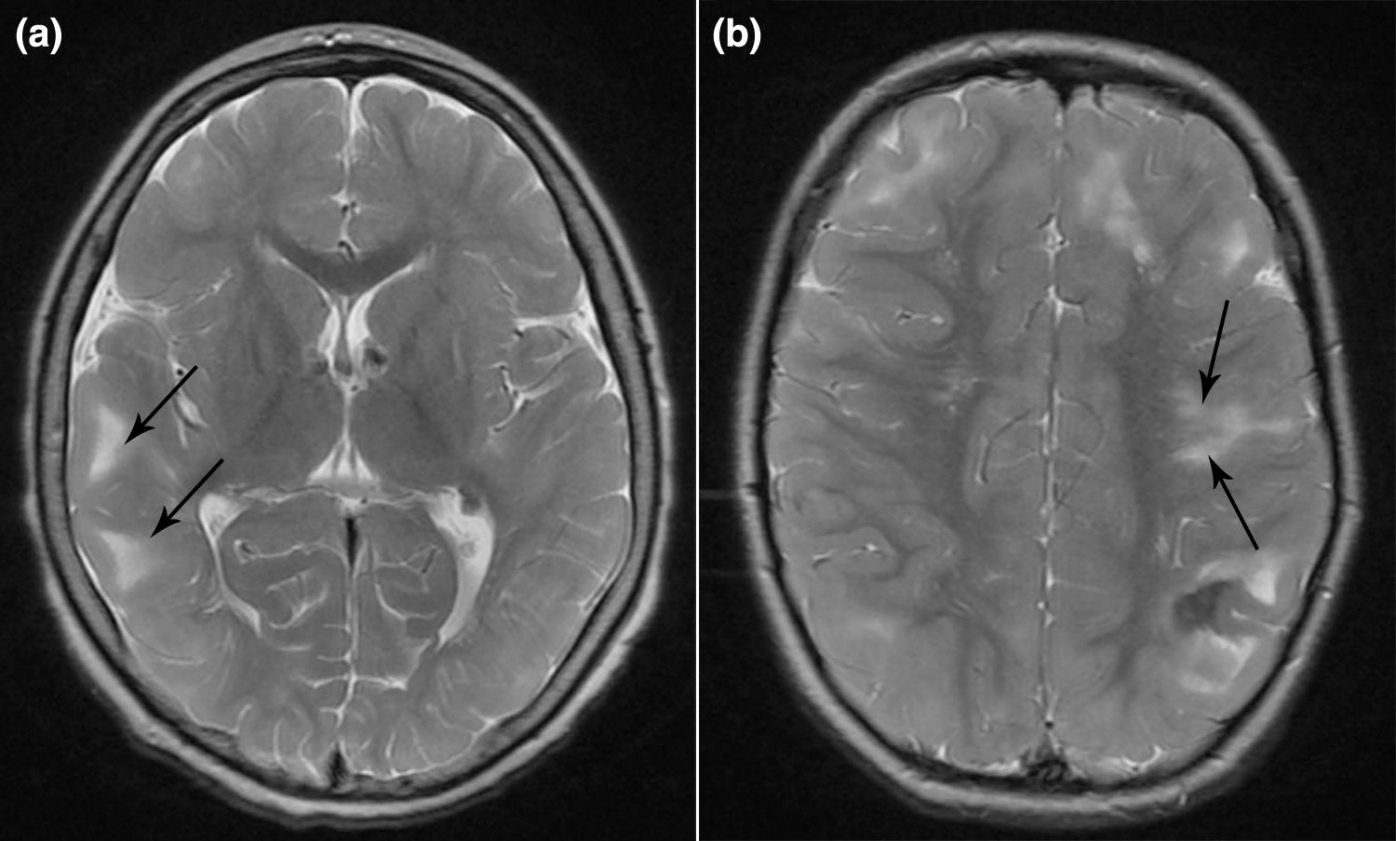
Example of T2-weighted images with arrows indicating a cortical tubers, and b radial migration lines
